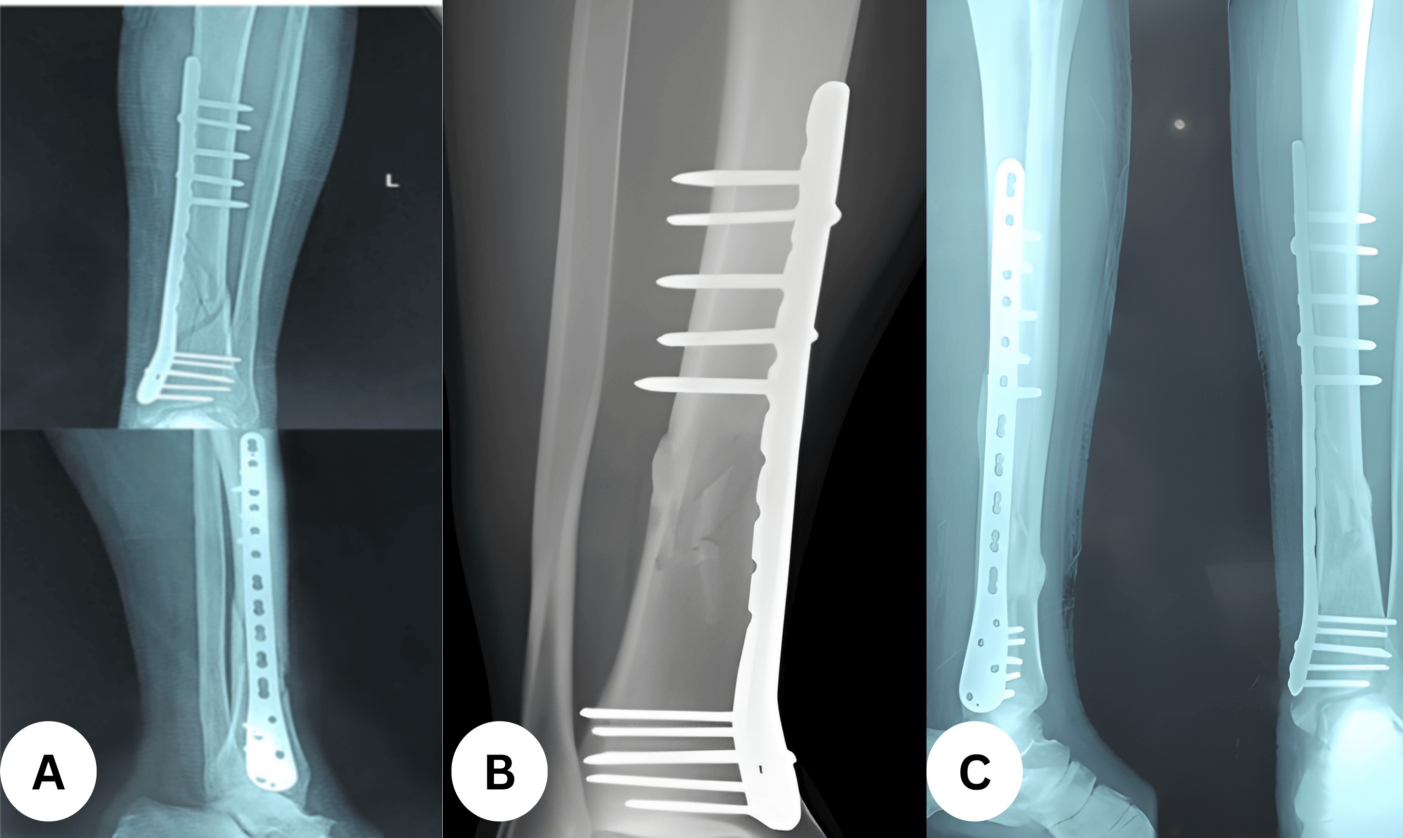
Serial follow-up after MIPO distal tibial locked plating (A) One-month radiograph showing maintained alignment with early callus formation; (B) three-month radiograph demonstrating progressive callus bridging; (C) six-month radiograph with cortical bridging consistent with union; hardware intact without loss of reduction. MIPO: minimally invasive plate osteosynthesis.
